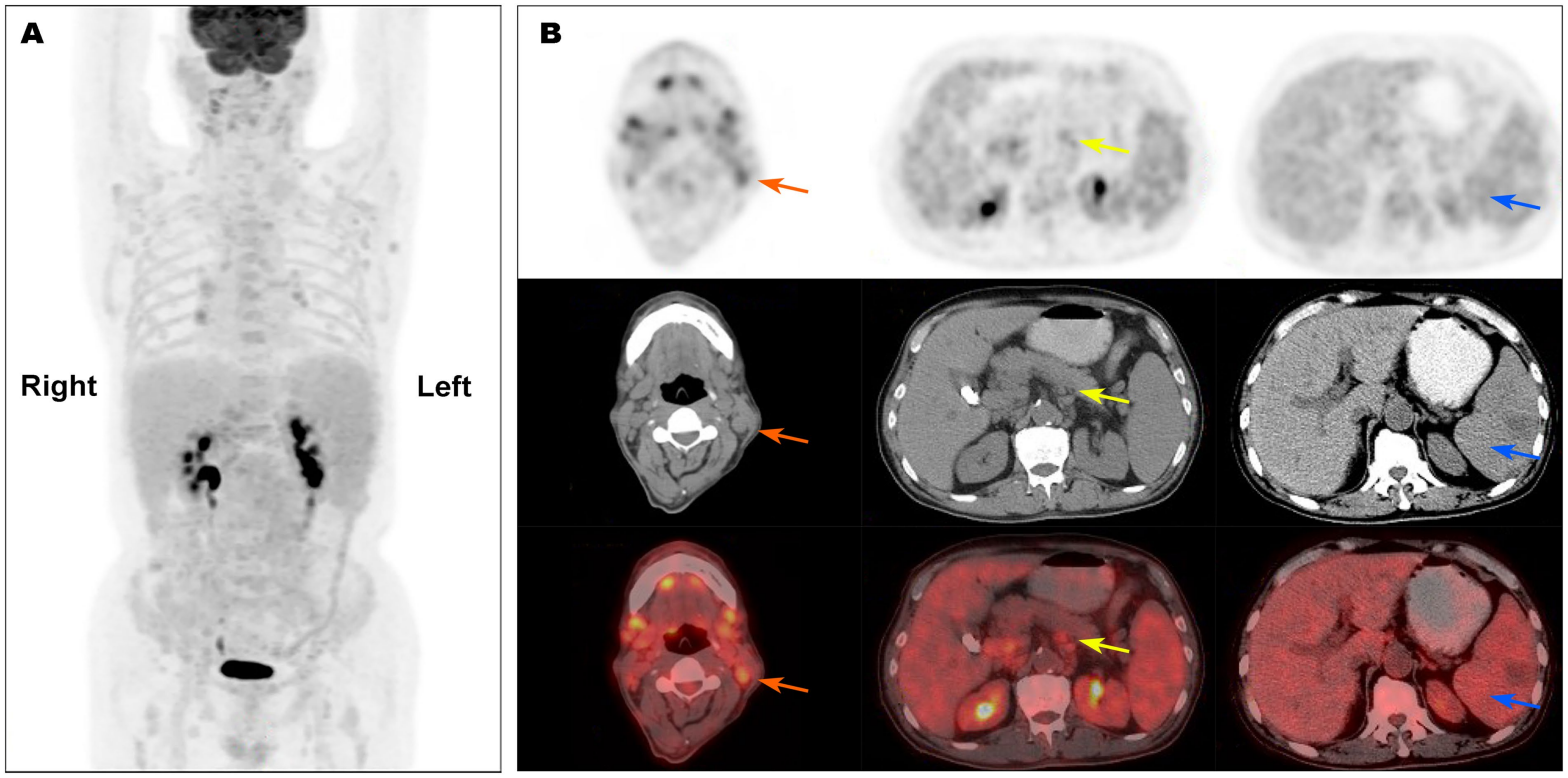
PET/CT scan showed multiple enlarged lymph nodes and splenomegaly. (A) showed enlarged lymph nodes above and below the diaphragm; (B) showed enlarged lymph nodes in cervical part (orange arrows) and abdominal part (yellow arrows), and splenomegaly (blue arrows).
